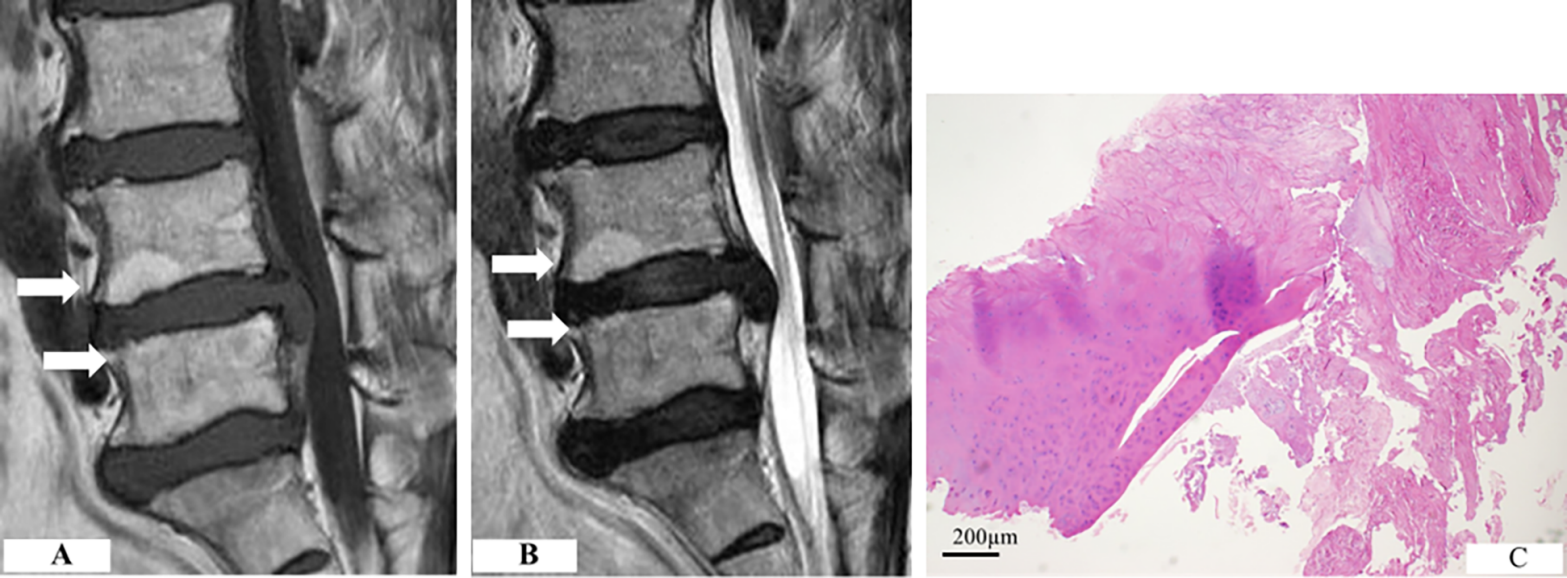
Cartilaginous herniation with Modic type 2 change. (A) The inferior endplate of L4 and the superior endplate of L5 show increased signal intensity on both T1-weighted scan. (B) The T2-weighted scan showed increased intensity in the same location. (C) Cartilaginous endplates were present in the herniated disc, along with the annulus fibrosus.
